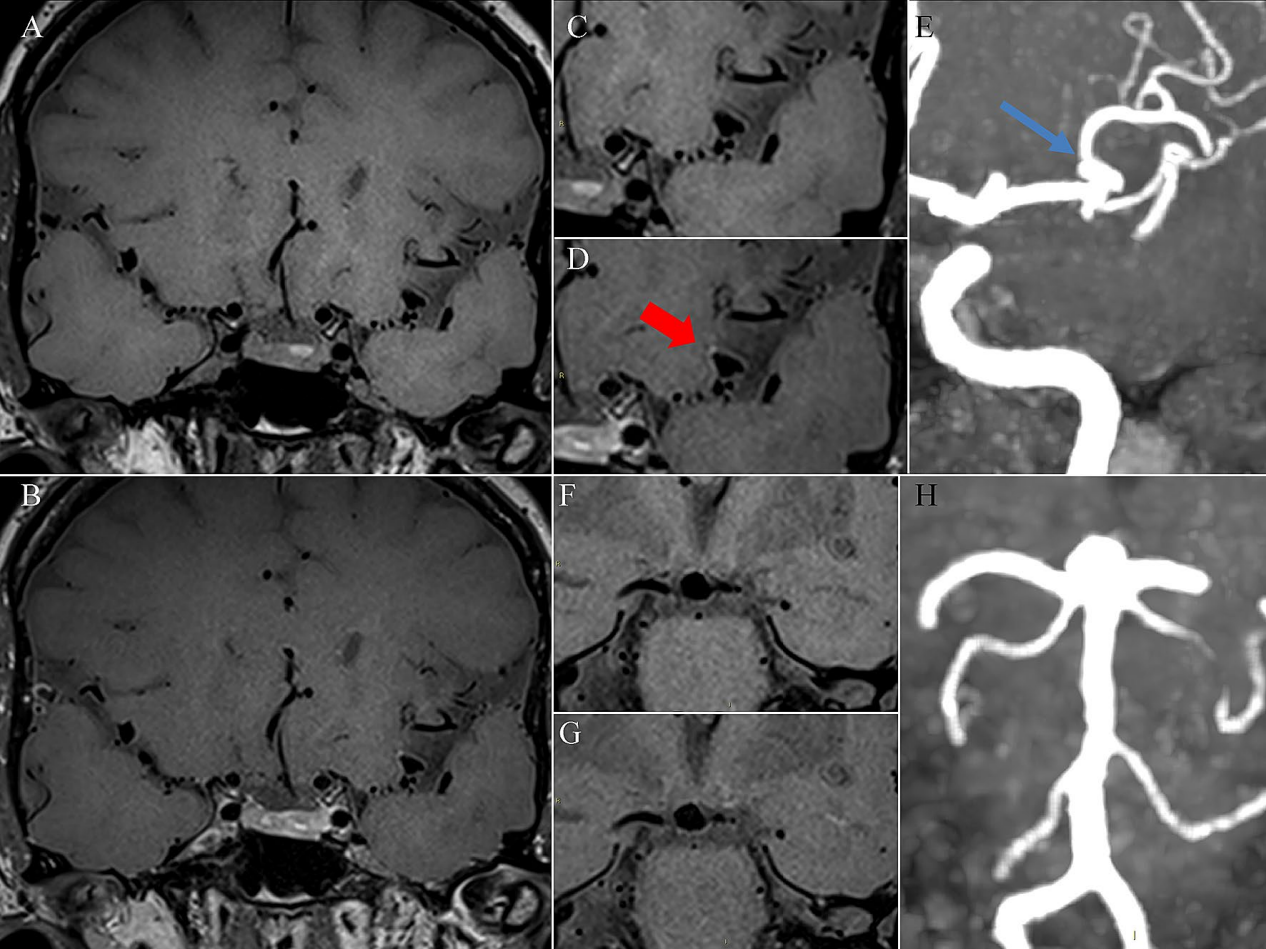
Aneurysms. A 64-year-old woman underwent brain MRI examination for the presence of incidentally discovering of multiple aneurysms, the largest located at left middle cerebral artery bifurcation (E) and at the basilar apex (H). Vessel Wall MRI sequences before (A) and after (B) the contrast medium administration, demonstrate the presence of a focal parietal enhancement after contrast medium of the left middle cerebral artery aneurysm (C, D red arrow, and E), while the basilar apex aneurysms did not show any parietal enhancement after the contrast medium administration (F, G, H). Despite the left bifurcation, MCA aneurysm did not fulfill the dimension criteria for treatment, the presence of wall enhancement let us decide to treat the aneurysm
